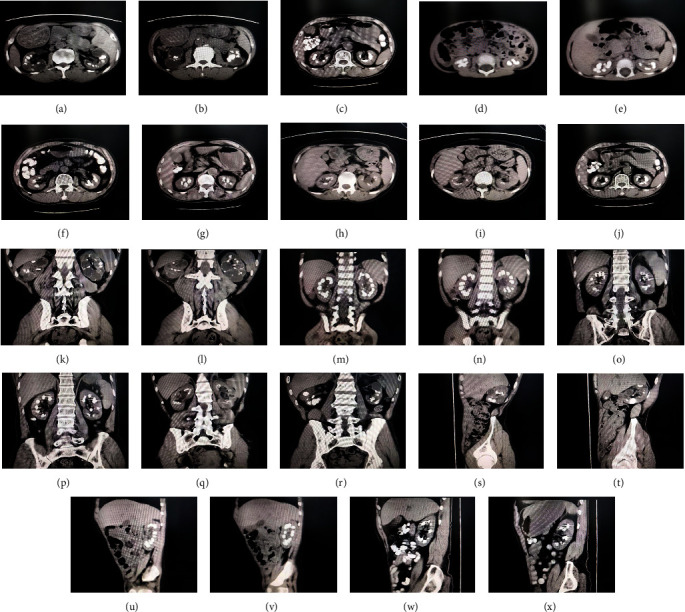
CT imaging features of MSK patients.
